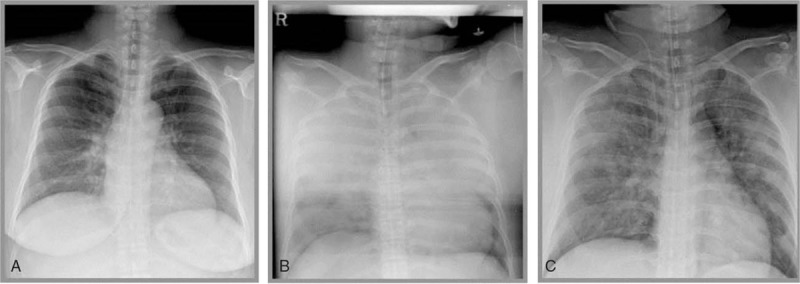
Changes in the chest radiograph findings. (A) Preoperative chest radiograph at 14 days prior to surgery. (B) Intraoperative chest radiograph. Opacification at the bilateral lung fields was notable, suggesting pulmonary edema. (C) Chest radiograph on postoperative day 1. Resolution of the pulmonary edema can be observed.
